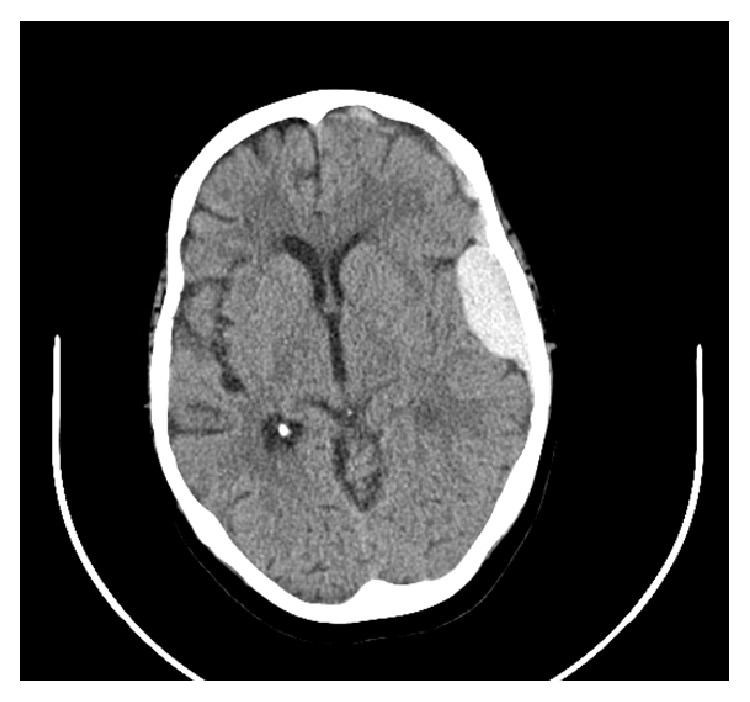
Initial axial CT head without contrast.
